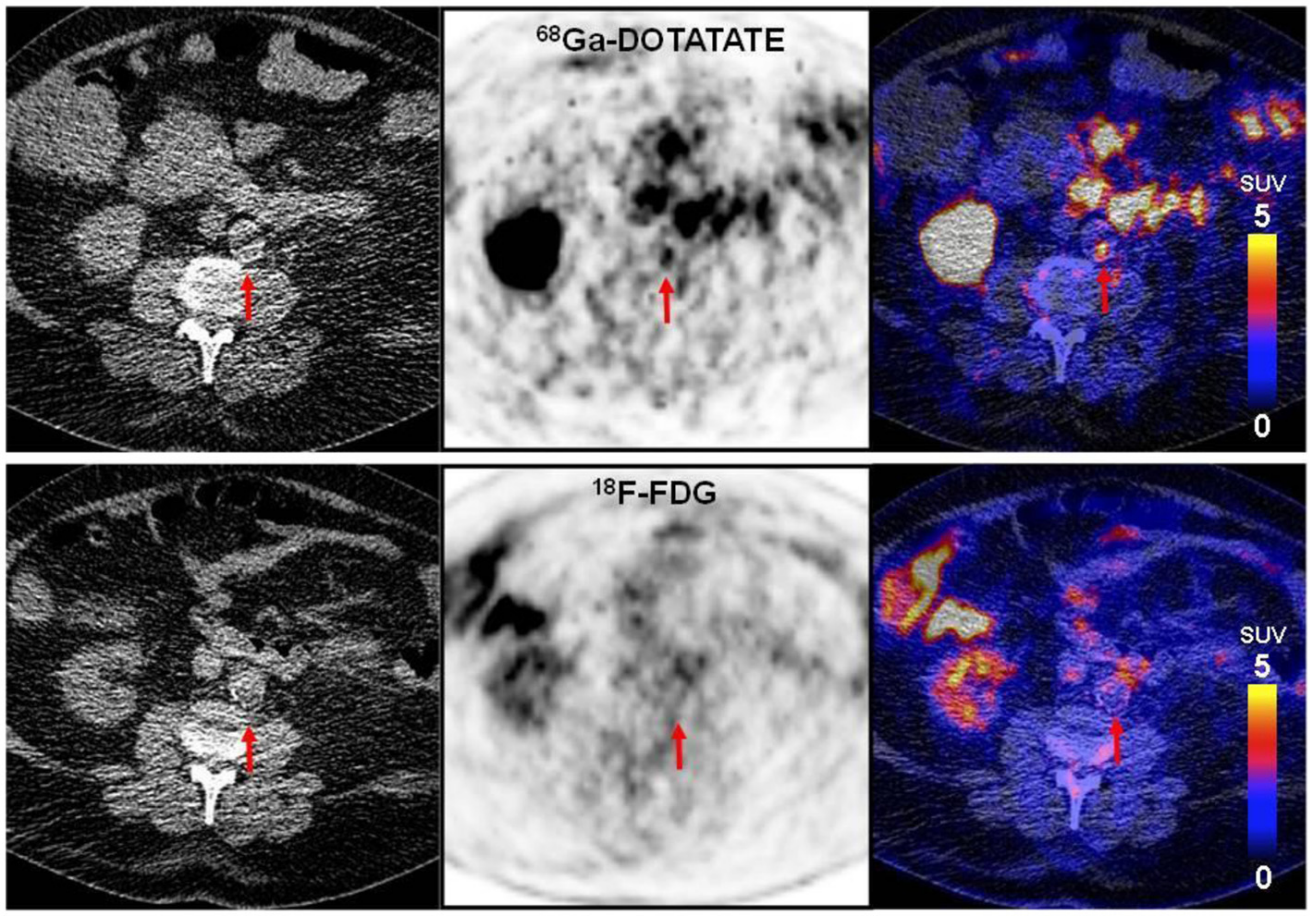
Focal vascular uptake of ^68^Ga-DOTATATE without corresponding focal ^18^F-FDG uptake. Transverse PET/CT images of a 73-year-old male patient with hypertension, hypercholesterolemia, and smoking. Intense focal uptake of ^68^Ga-DOTATATE can be observed in the aortic arch (upper row), whereas no focally increased ^18^F-FDG uptake was seen (lower row). TBR_DOTATATE_ was 7.60, while TRB_FDG_ was 1.74.
